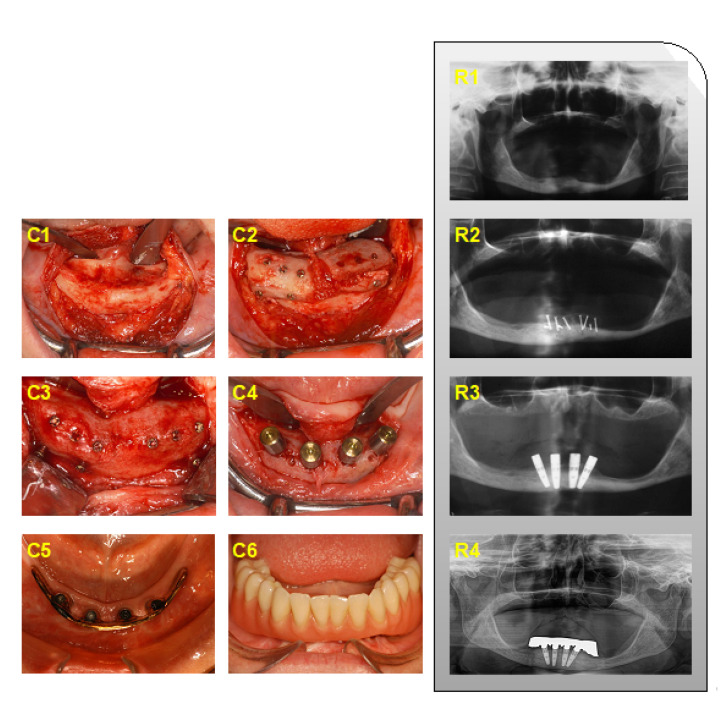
Clinical (C1–C6) and radiographic (R1–R4) presentation of iliac crest bone augmentation in the interforaminal region. (C1) Intraoperative situs; (C2) fixation of corticocancellous bone blocks; (C3) removal of osteosynthesis screws after healing; (C4) implant placement, (C5,C6) at the time of prosthetic reconstruction with; (C5) suprastructure with a milled bar; and (C6) final prosthesis in situ. (R1) Preoperative situation, (R2) after iliac crest bone augmentation, (R3) after implant placement, and (R4) follow-up after 11 years.
